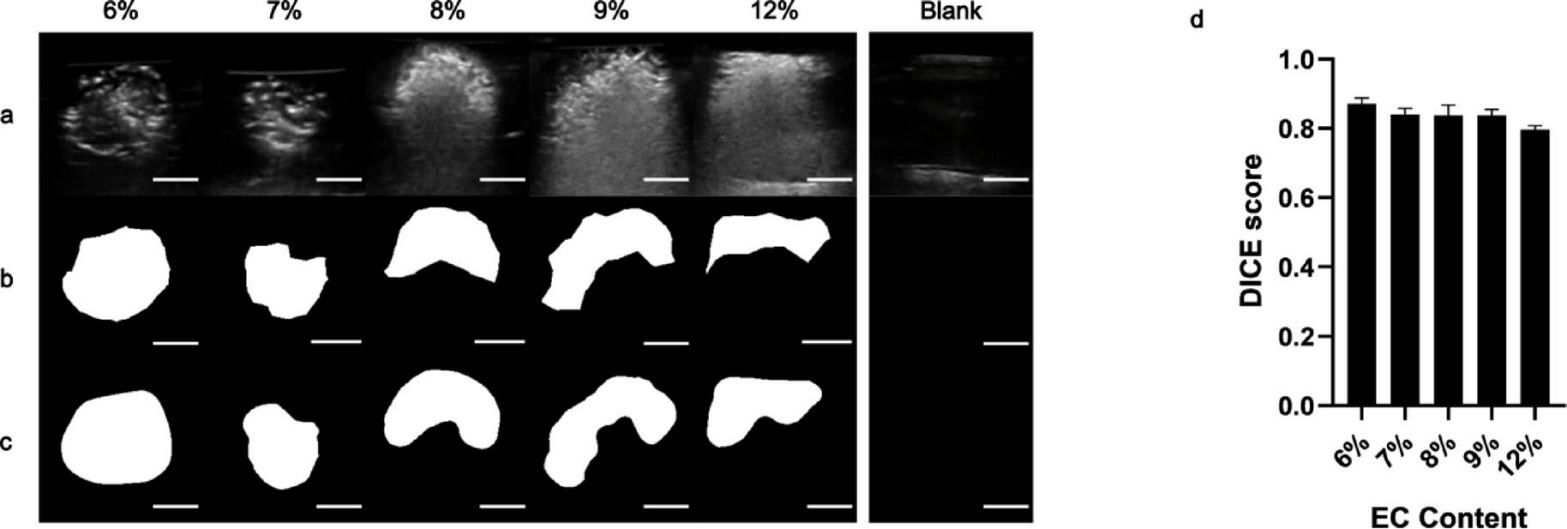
Visibility of different concentrations of EC-ethanol in agar under ultrasound imaging. (a) Representative B-mode images and binary masks of the EC-ethanol depots generated via (b) manual segmentation and (c) automatic segmentation. Scale bars = 5 mm. (d) Dice scores for measuring the similarity between binary masks generated from manual depot segmentation vs. automatic segmentation. *N* = 10.
